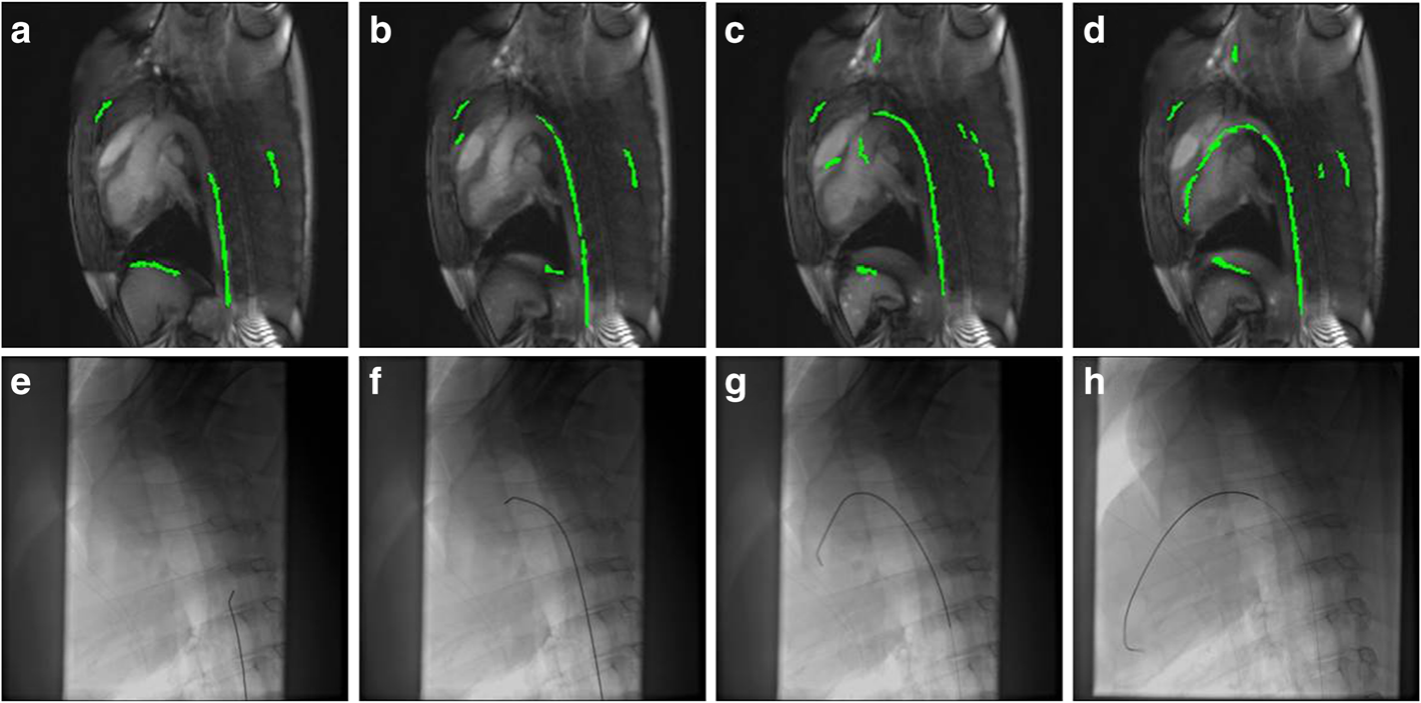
Left heart catheterization images using dual echo CMR guidance and X-Ray guidance. Example images during left heart catheterization under CMR-guidance (a-d) and X-Ray guidance (e-h). Images are taken at the identical location indicated by markings on the guidewire when the tip is in the descending aorta (a, e), at the aortic arch (b, f), at the aortic valve (c, g) and in the left ventricle (d, h).
